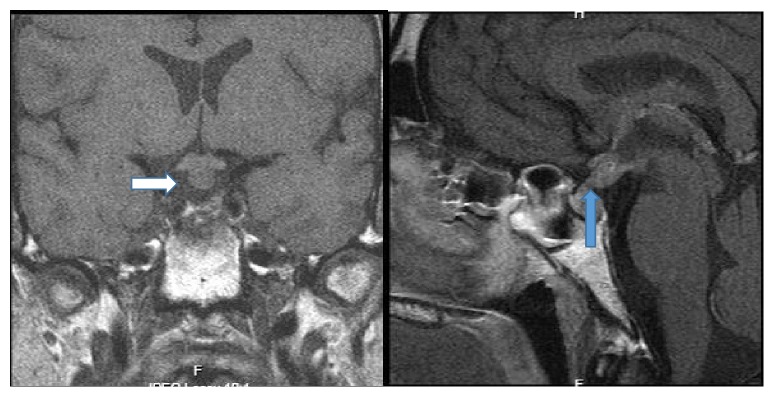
Magnetic resonance imaging (MRI) with gadolinium. A: T1-weighted showing diffuse thickening of the infundibulum (white arrow). B: T1-weighted sagittal showing absence of the posterior pituitary bright spot (blue arrow).
